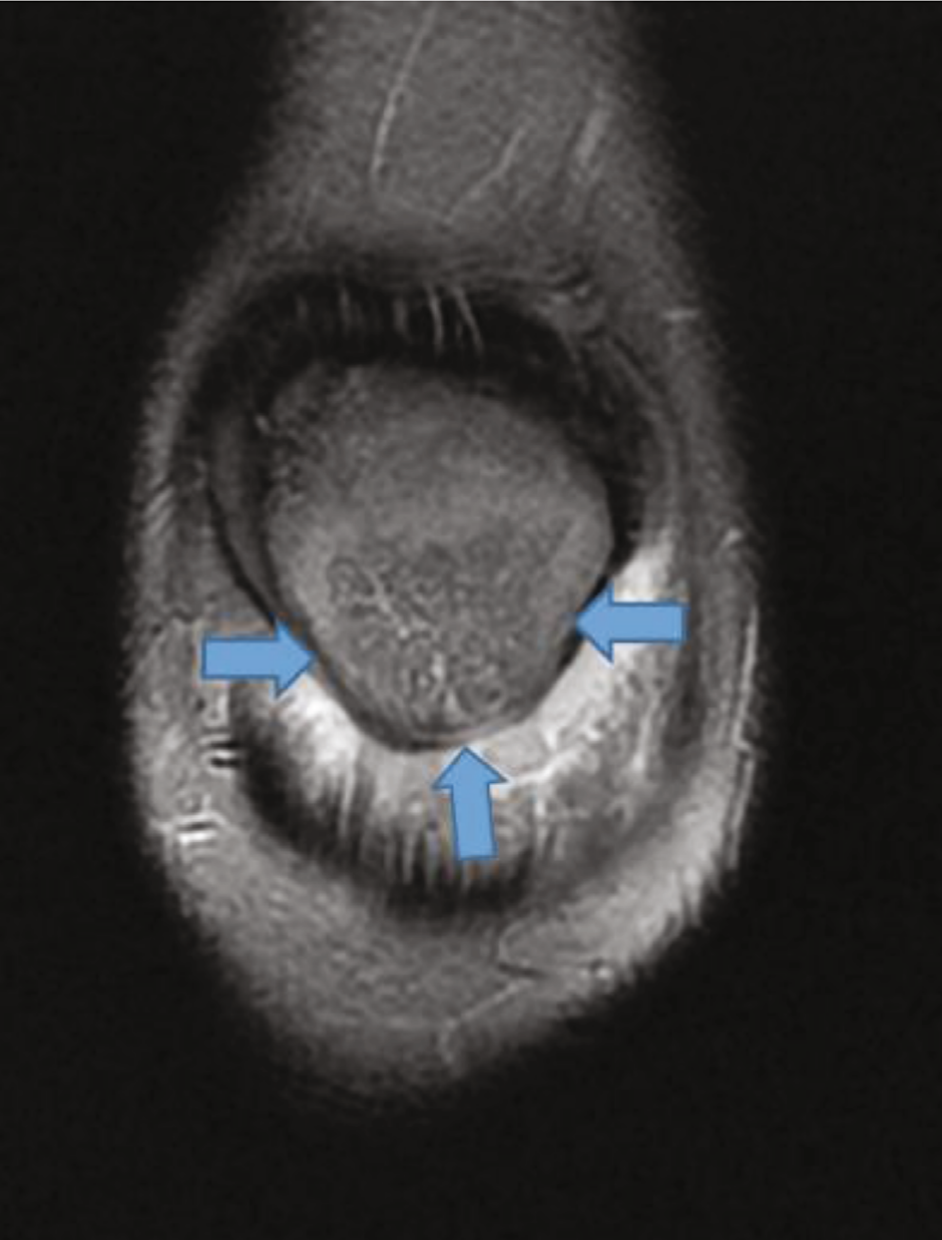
MRI 2. Coronal T2 fat sat view. Osteomyelitis of the patella (blue arrows).
